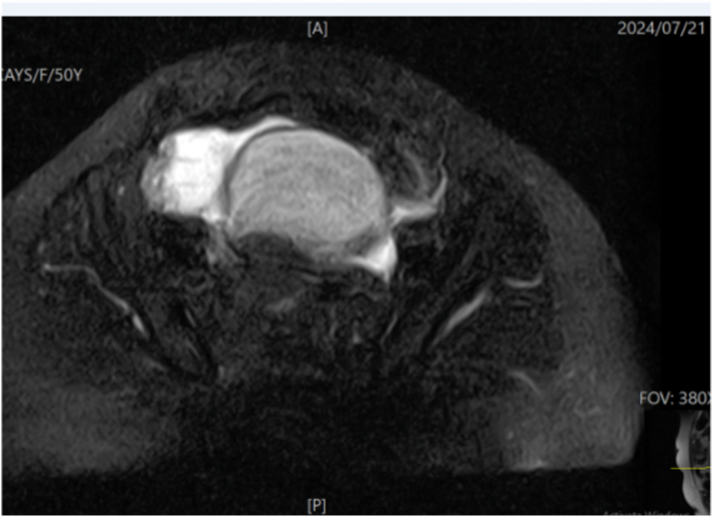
MRI findings demonstrating a benign-appearing pelvic lesion on the right ovary, most likely a cystadenoma, accompanied by pelvic inflammatory disease with significant localized fluid collection in the pouch of Douglas.
